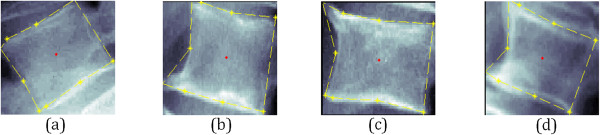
Examples of AOs classes of the lumbar x-ray images. The four classes of AOs in lumbar vertebrae (a) normal (b) L-LHC (c) U-LHC and (d) B-LHC.
